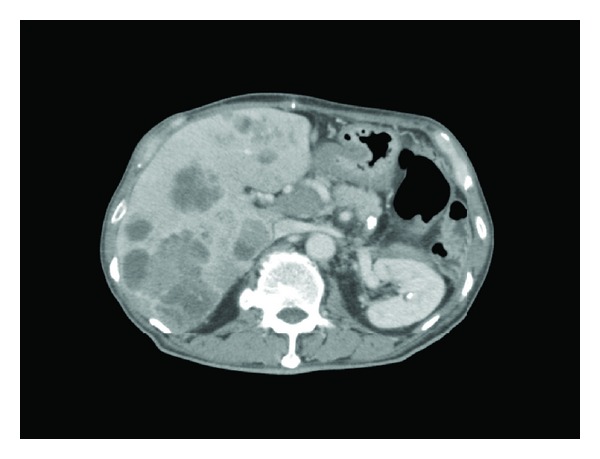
Abdominal computed tomography at the time of recurrence. Multiple liver metastases with portal thrombosis were found 8 weeks after surgery.
